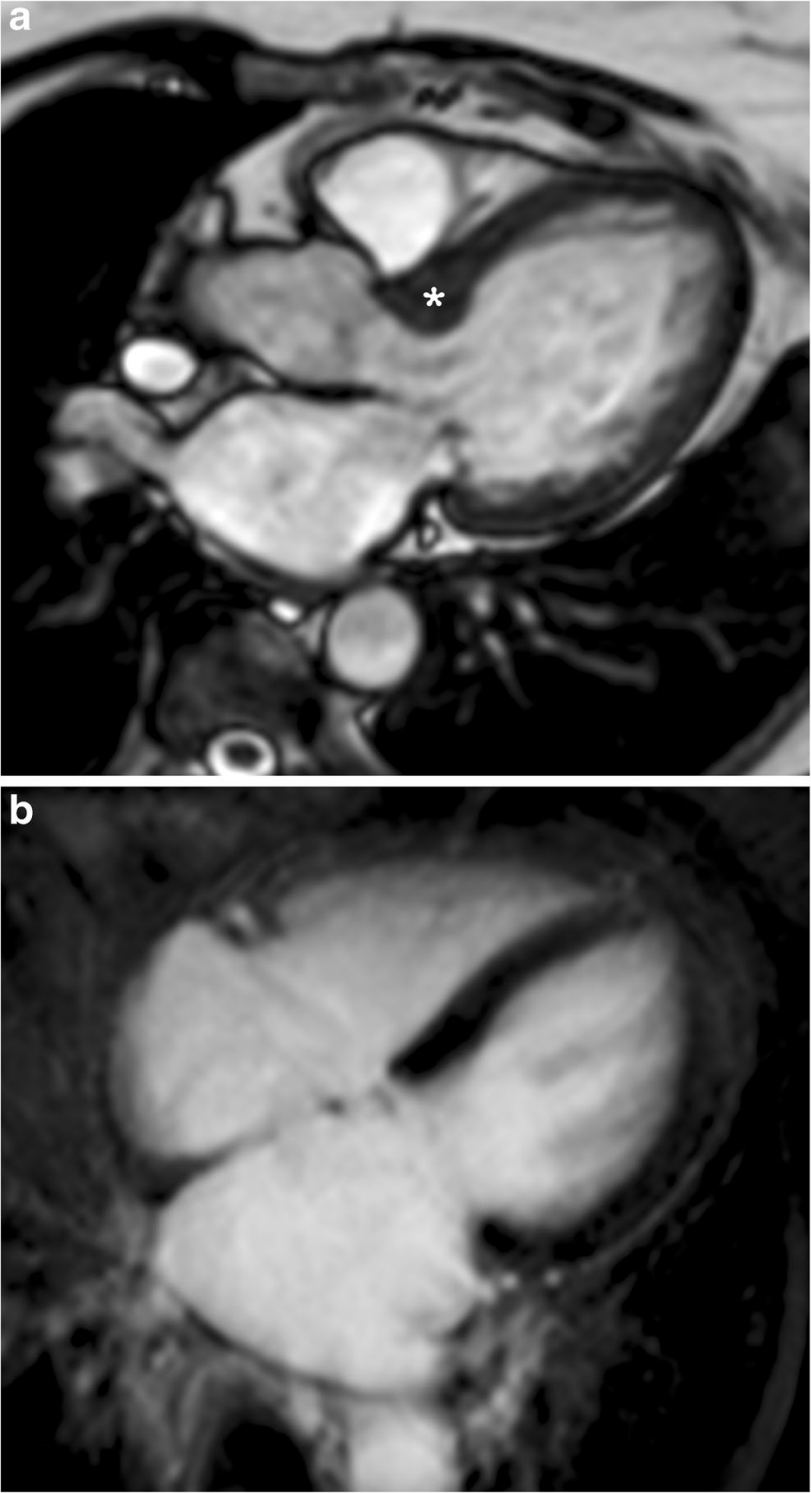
A 64-year-old woman with essential hypertension treated for several years. a Three-chamber cine SSFP MR image at end-diastole showing asymmetrical basal septal myocardial hypertrophy (*asterisk*). b Four-chamber view of LGE image shows normal dark myocardial signal intensity. Left atrial enlargement also can be seen
